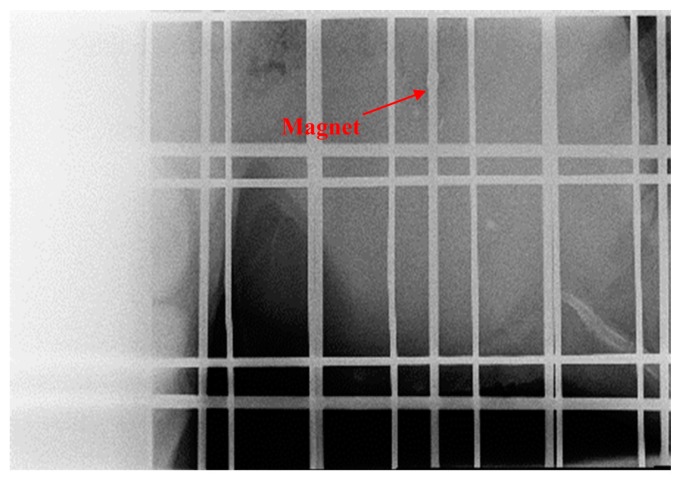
Location of the magnet is verified by X-Ray.
